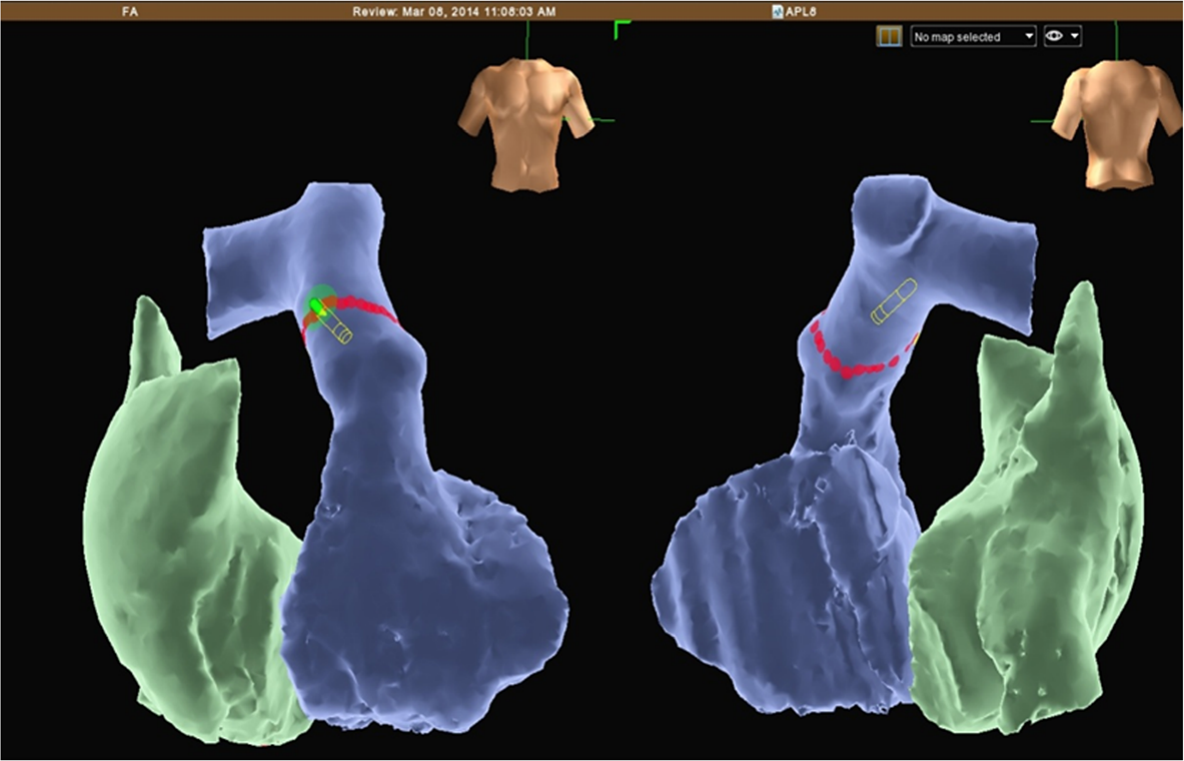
Electroanatomic reconstruction of the right ventricular outflow tract and pulmonary artery was performed using the EnSite Velocity Cardiac Mapping System, and a merger was made with a formatted image obtained by performing cardiac computed tomography angiography
